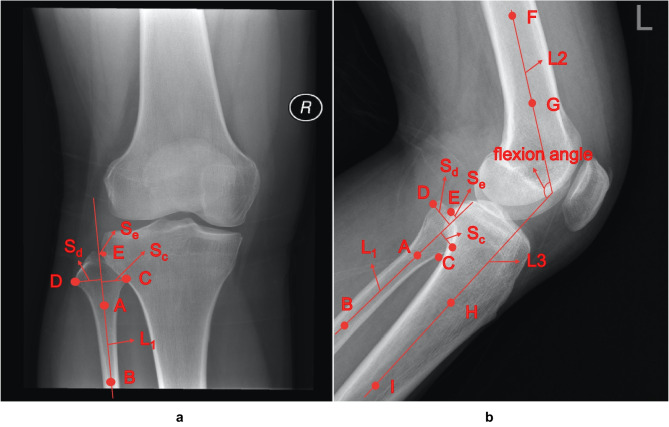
Example annotations of predefined key points and their corresponding auxiliary lines. a AP knee plain radiograph. b LAT knee plain radiograph. Auxiliary lines L1, L2, L3, vertical lines Sc, Sd, Se and flexion angle are all shown
